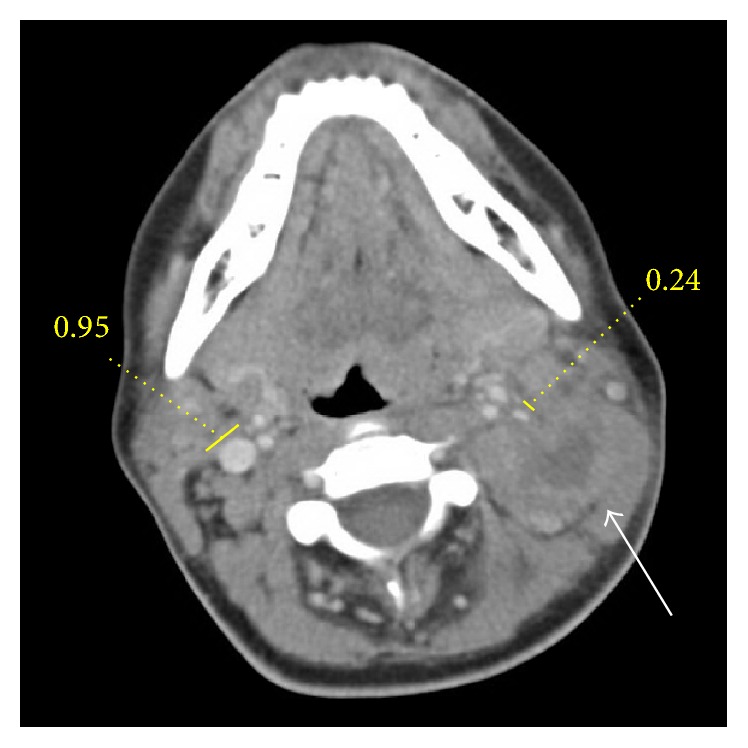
Computed tomography scan of the neck without contrast showing enlarged bilateral lymph nodes. On the patient's left, the largest lymph node (4.1 × 2.7 cm; white arrow) has a central hypodensity (1.9 × 1.2 cm) consistent with fatty necrosis. Additionally, the right internal jugular (IJ) vein measured 0.95 cm in the greatest diameter whereas the left IJ vein was 0.24.
